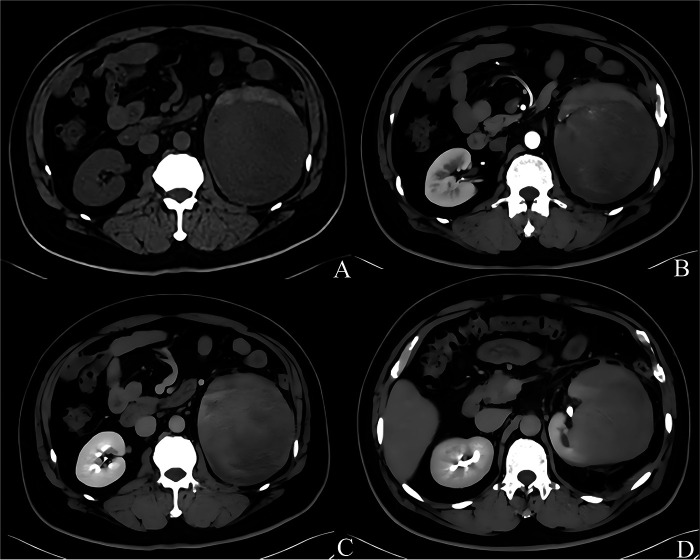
CT of both kidneys [panels (A–D) correspond to the unenhanced phase, arterial phase, venous phase, and delayed phase, respectively].
